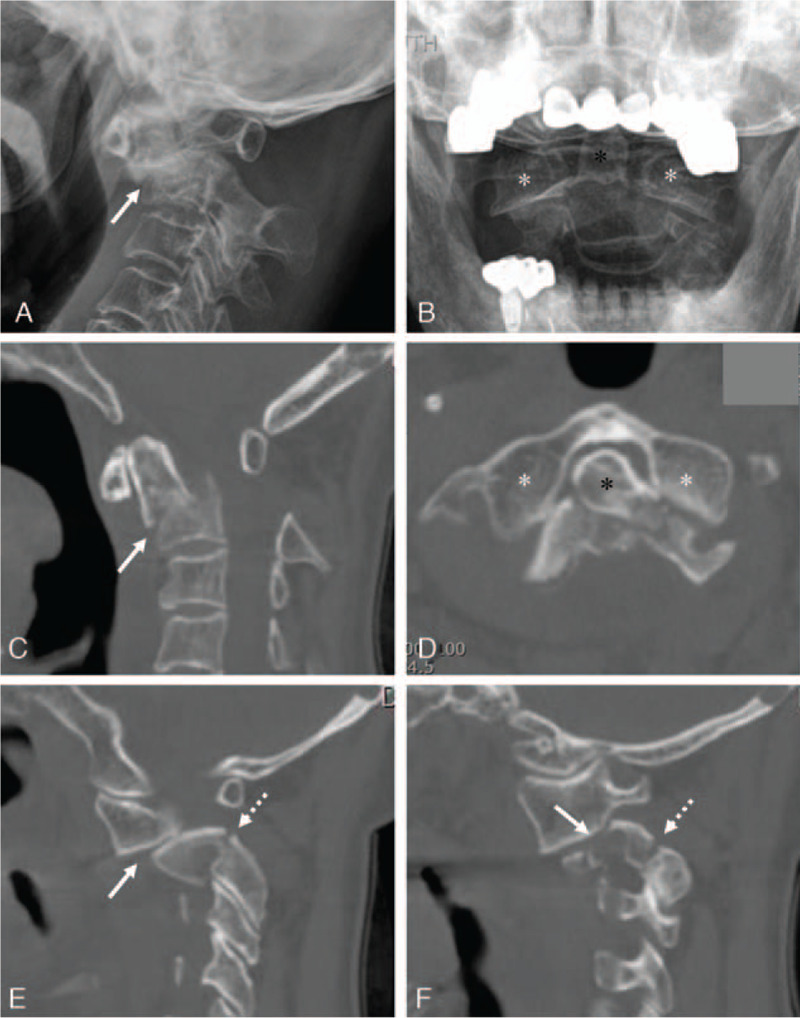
A lateral radiograph (a) and midline sagittal computed tomography (CT) (c) scan revealed the anteroinferior displacement of a type IIA dens fracture with kyphosis (white arrow). An open-mouth view (b) and axial CT scan (d) revealed an atlantoaxial anteroinferior subluxation (white asterisks) and anterolateral rotatory angulation of the type IIA dens fracture fragment (dark asterisk). The right (e) and left (f) parasagittal CT scans revealed fractures of both pars interarticularis (type I Hangman fracture) (dotted white arrows) and the left transverse foramen (white arrow).
